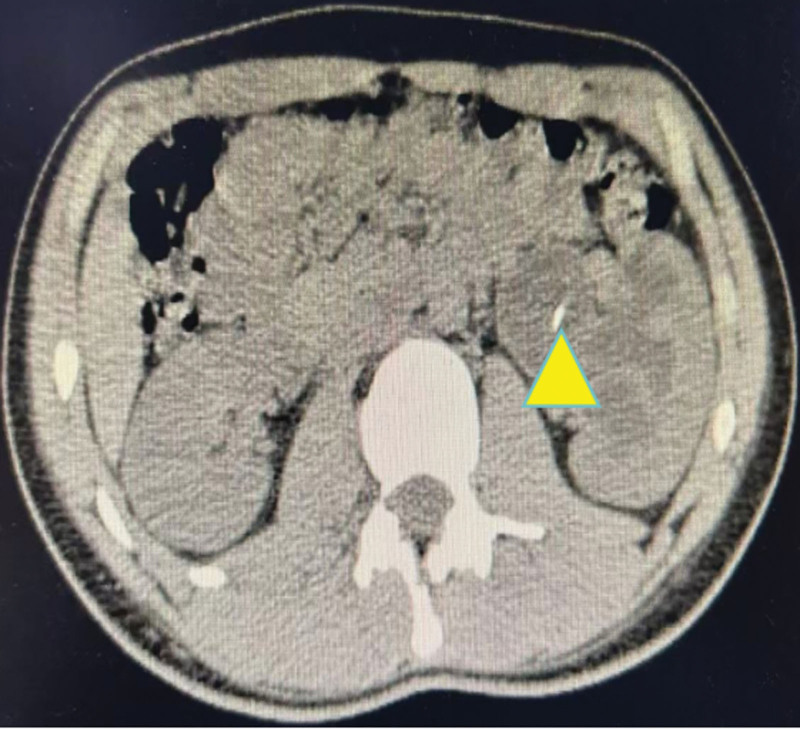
Computed tomography urography. Left renal hydronephrosis (indicated by yellow triangle).
